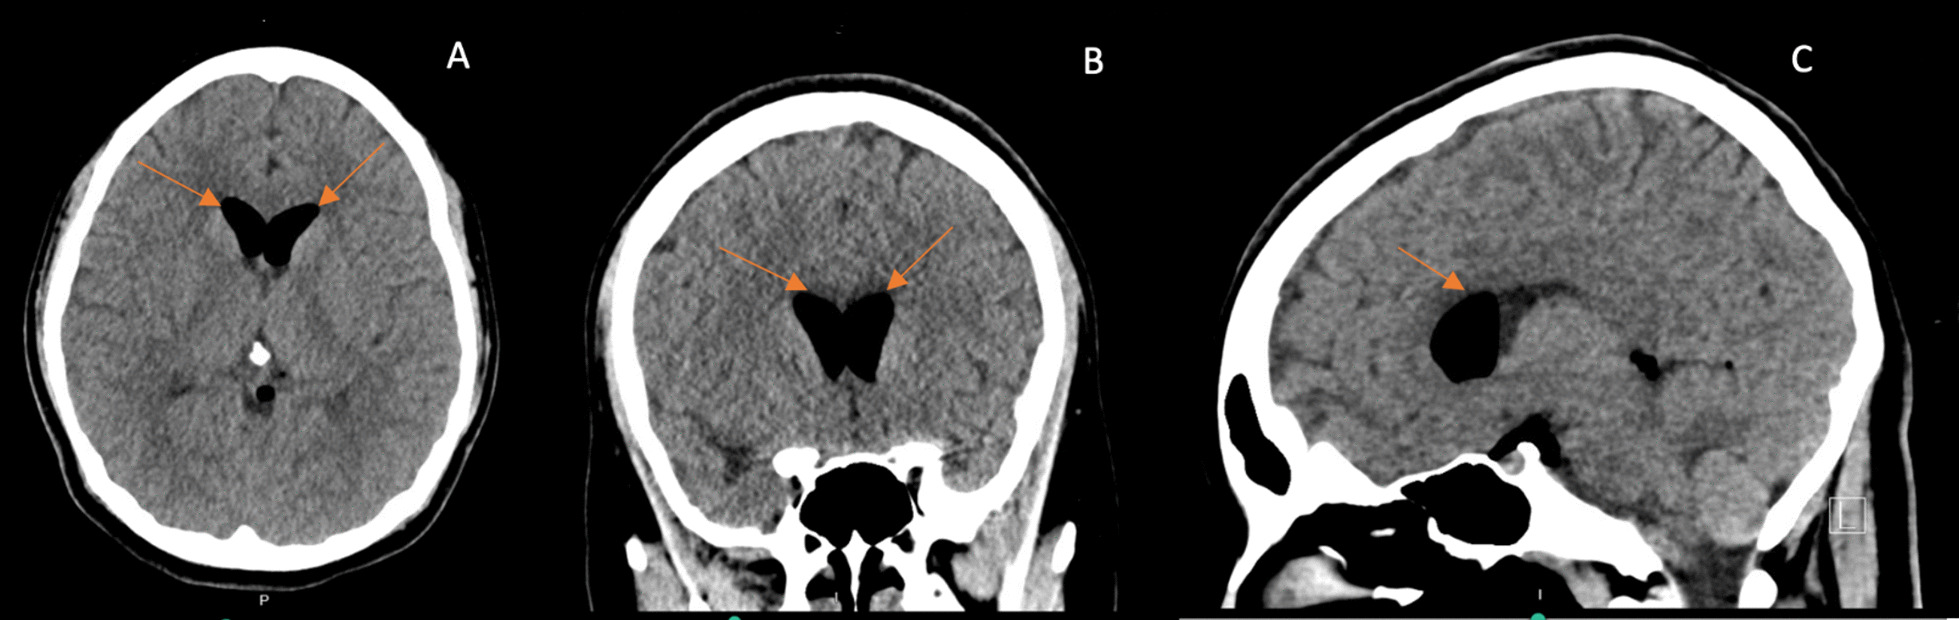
Computed tomography head showing air predominantly within the frontal horn of the lateral ventricles without midline shift or mass effect. A Axial view, B Coronal view, C Sagittal view. Orange arrows identify the abnormal presence of air
